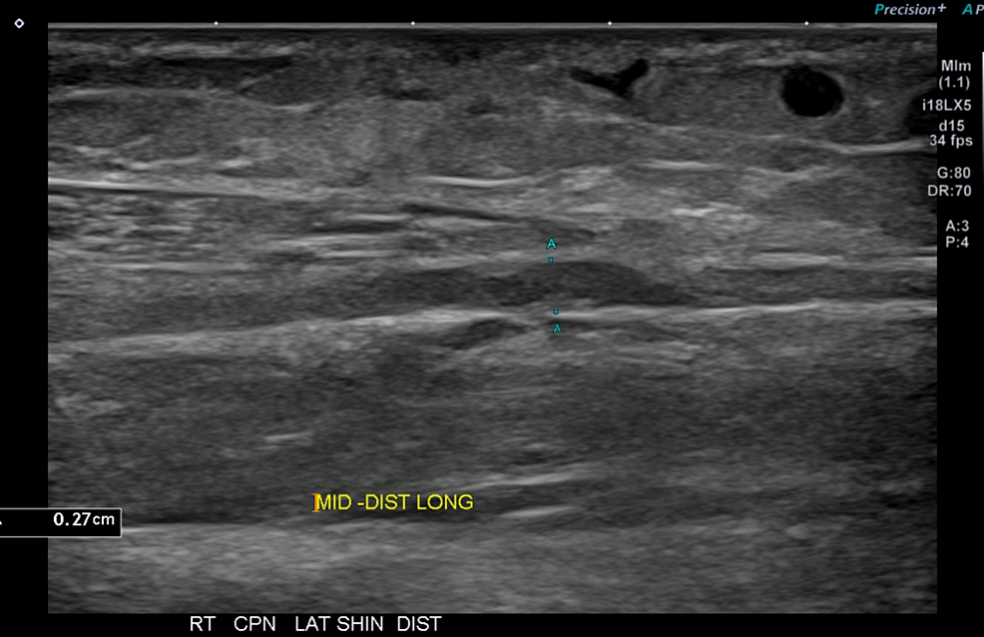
Longitudinal ultrasound image showing a neuroma-in-continuity of the thick superficial branch of the right common peroneal nerve.
